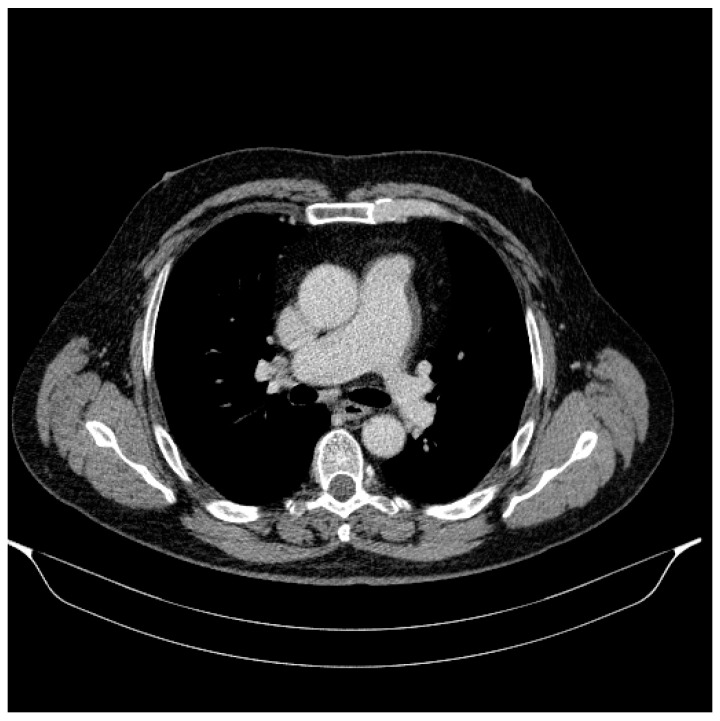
The computed tomography of the chest (September 2023): no lesions with a suggestive appearance for secondary thoracic determinations.
